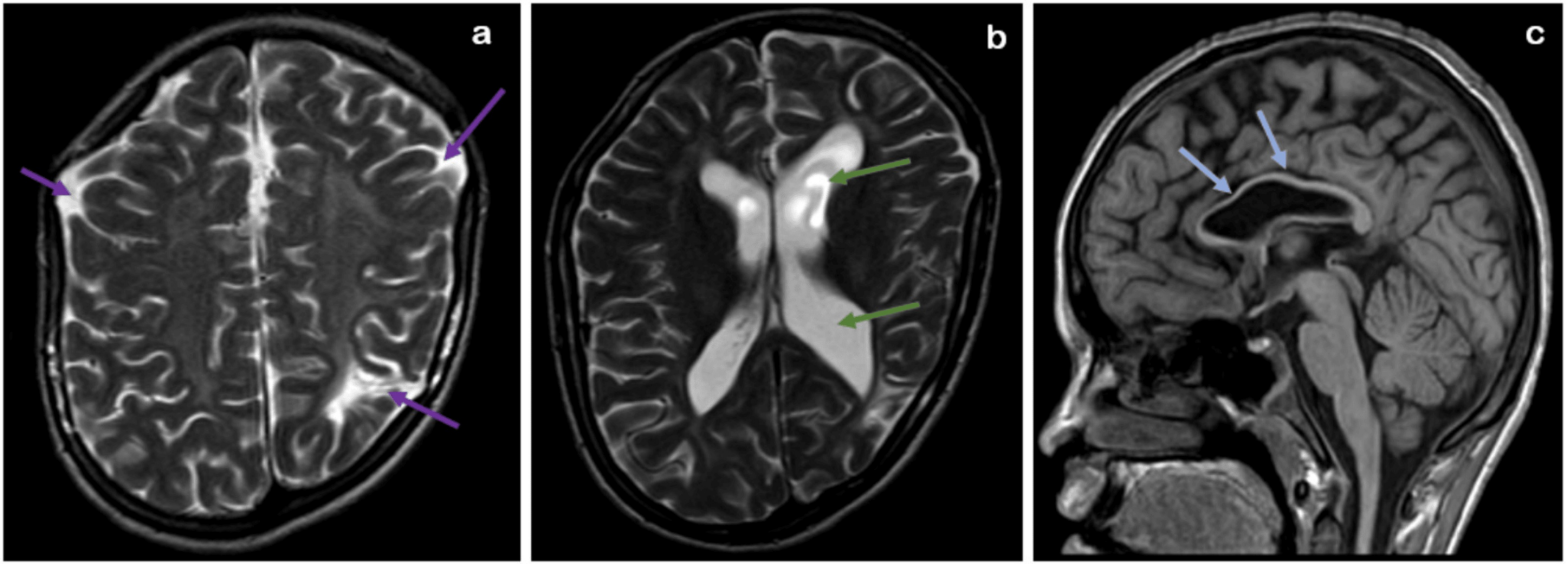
MRI brain T2 sequence axial images (a,b) demonstrate gliosis with encephalomalacic changes in the bilateral frontal and left parietal lobes (violet arrows), dilated left lateral ventricle (green arrows) and (c) T1 sequence coronal image shows a thinned genu and body of the corpus callosum (blue arrows).
